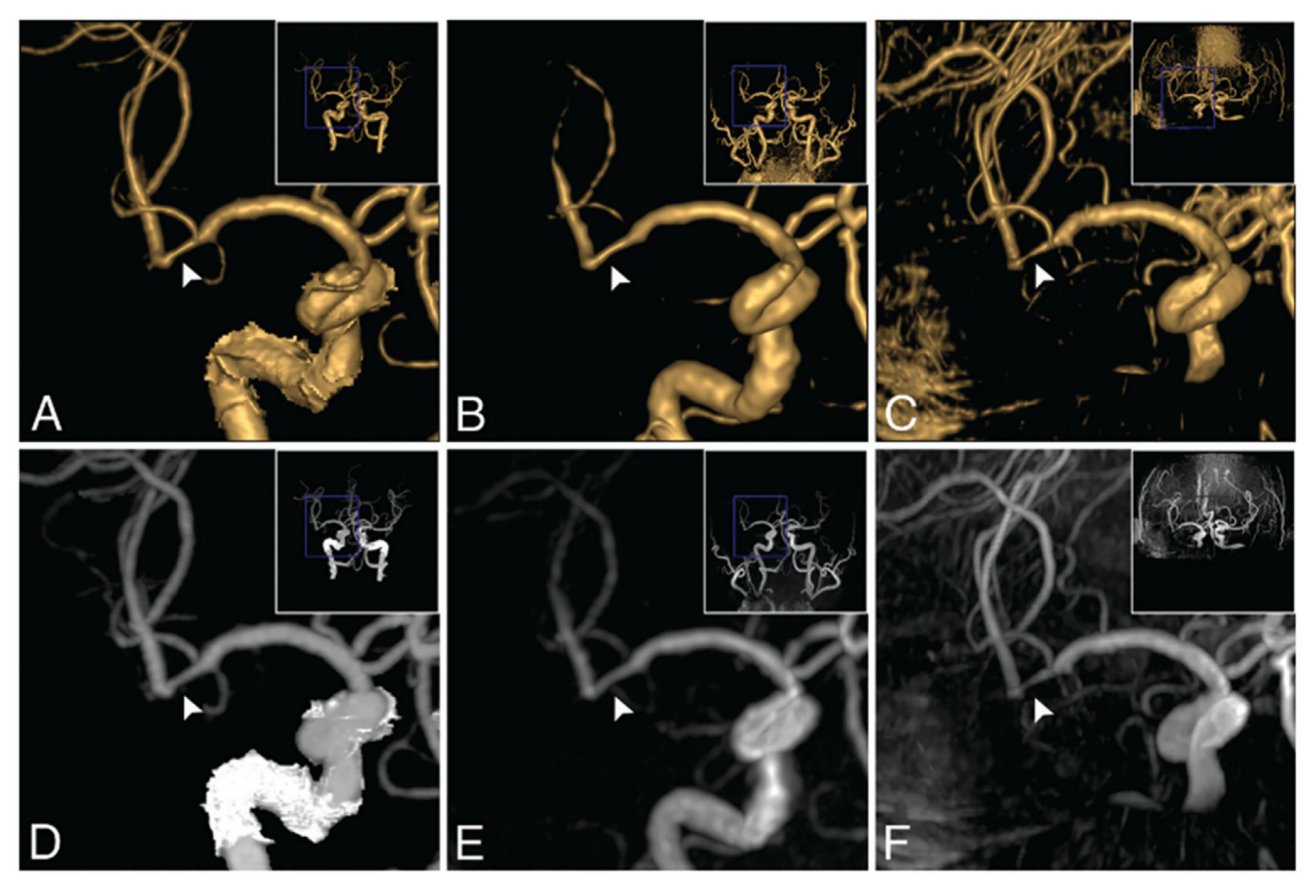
Example of ZTE-MRA (B and E) compared to computed tomography angiogram (CTA) (A and D) and TOF (C and F). Top row shows volume rendering and bottom row maximum intensity projection. Image shows a stenosis in a 74-year-old male patient. CTA estimated a 34% stenosis, ZTE estimated 32%, while TOF overestimated the stenosis to 72%. Republished with permission of American Society of Neuroradiology from Ref. [68]; permission conveyed through Copyright Clearance Center, Inc.
